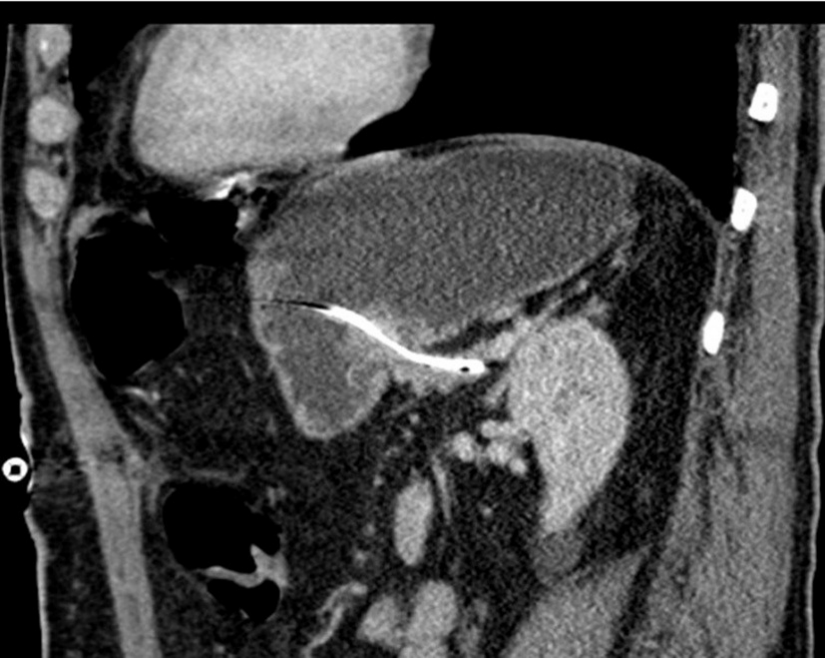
CT image of pancreatic duct drainage in the pancreatic stump and stomach.
